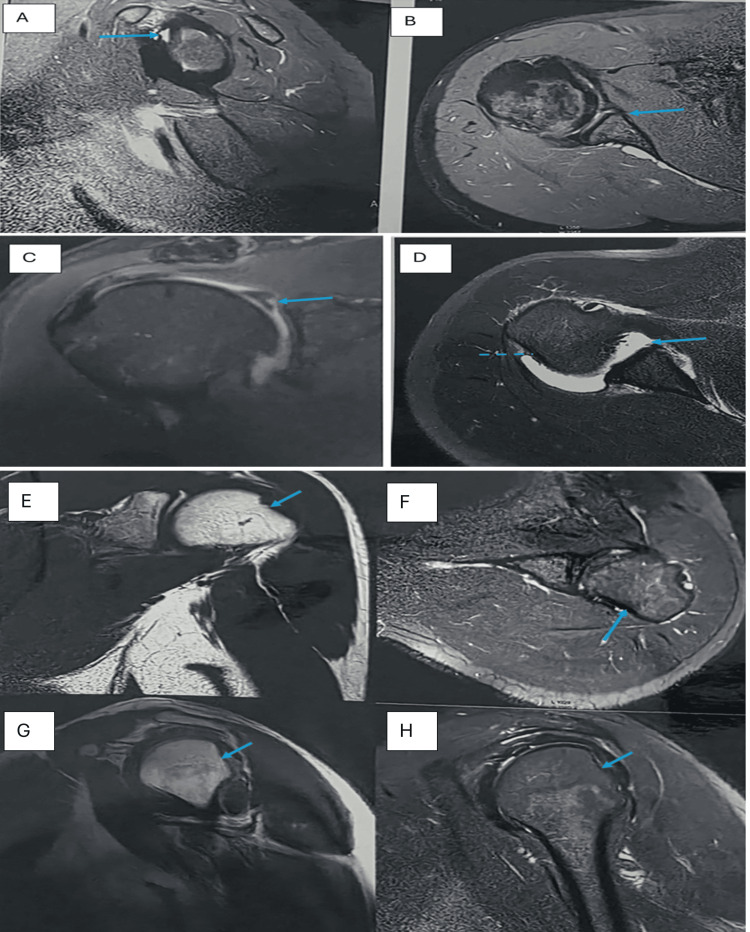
Labral and instability-related lesions on MRI PDFS images: (A-B) ALPSA lesion showing linear hyperintensity along the periosteum of the anterior glenoid. The labrum appears intact. (C) Type 2 SLAP lesion showing linear hyperintensity in the superior labrum. (D) Bankart lesion with attenuation and hyperintensity in the anteroinferior glenoid labrum (solid arrow) and associated cortical flattening of the posterosuperior aspect of the humeral head (dotted arrow), suggesting Hill-Sachs deformity ALPSA, anterior labroligamentous periosteal sleeve avulsion; SLAP, superior labrum anterior to posterior; PDFS, proton density-weighted fat-saturated; MRI, magnetic resonance imaging
